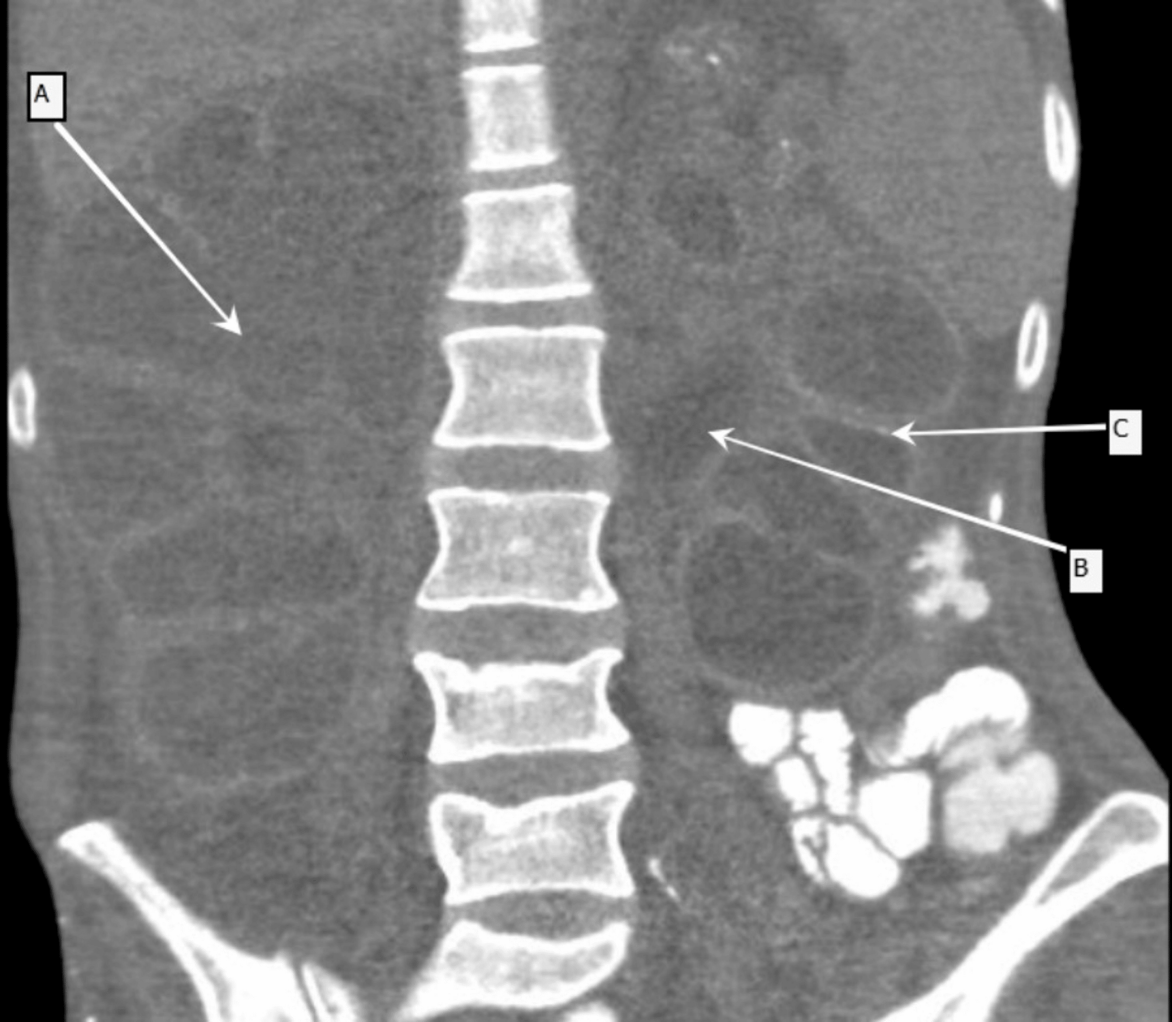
Non-contrast computed tomography of the abdomen Coronal section showing markedly enlarged bilateral renal parenchyma with dilated calyces [A], left-sided severe hydronephrosis [B], and calcifications in the junctions of the corticomedullary junctions of the kidneys bilaterally [C].
